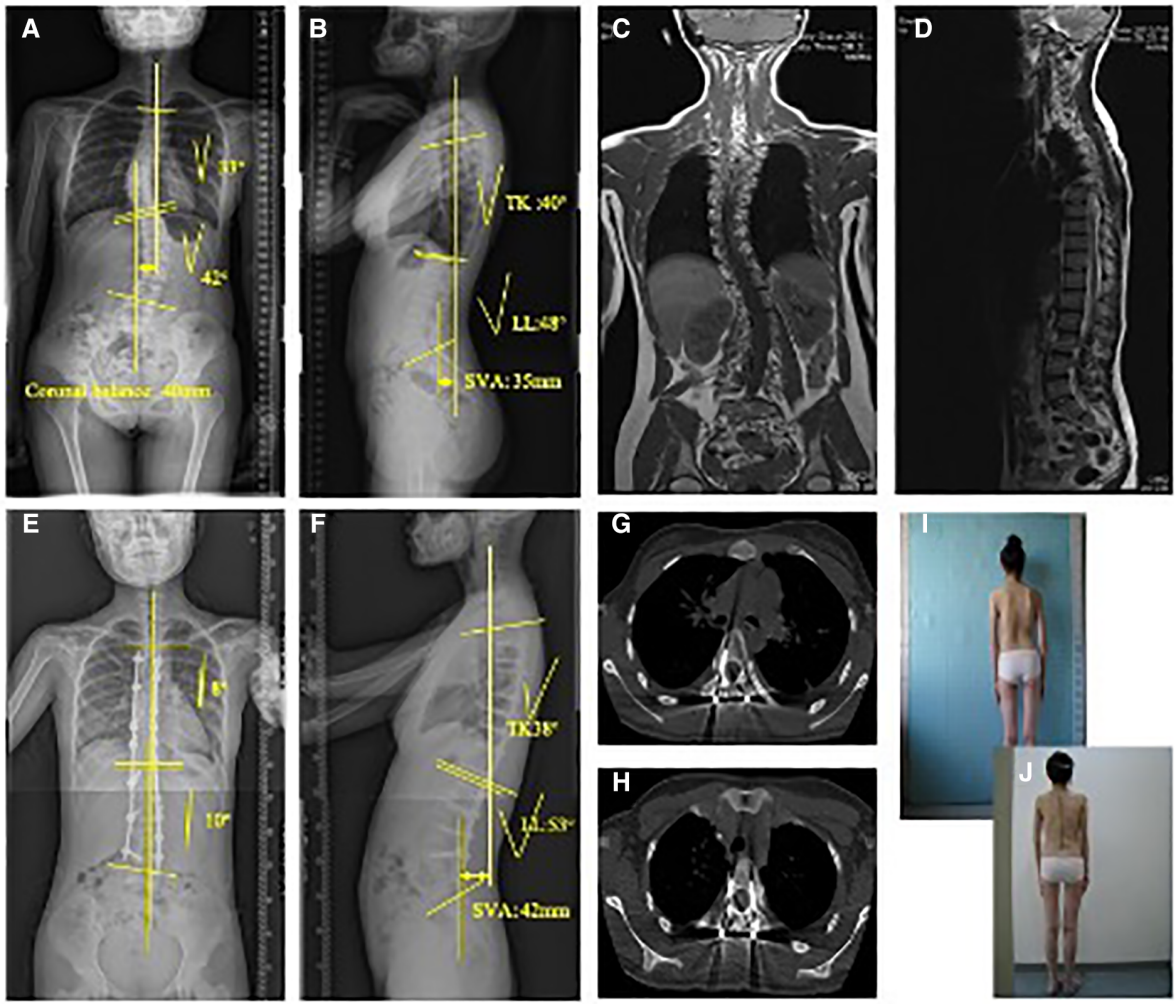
This picture showed the clinical pre-operative picture of this scoliosis patient. A 14-year old female Lenke 1 AIS patient underwent robot-assisted scoliosis correction surgery. Preoperative anteroposterior (A) and lateral (B) full spine X-ray showed a Cobb angle of 42°in major thoracic curve and 33°in secondary lumbar curve (the angle between major curve and secondary curve). Coronal (C) and sagittal (D) view of full spine MRI showed there was no abnormalities within spinal cord, which supported the diagnose of AIS when combined with other clinical characteristics. Postoperative anteroposterior (E) and lateral (F) full spine X-ray showed major thoracic curve was corrected to 10°, while secondary curve was corrected to 8°. Postoperative CT scan was used to evaluate the accuracy of screw insertion, and the typical image of satisfactory position (G) and lateral cortical perforation (H) were shown. In addition, clinical images of the patients both pre-operatively (I) and post-operatively (J) were also shown in Figure 3.
